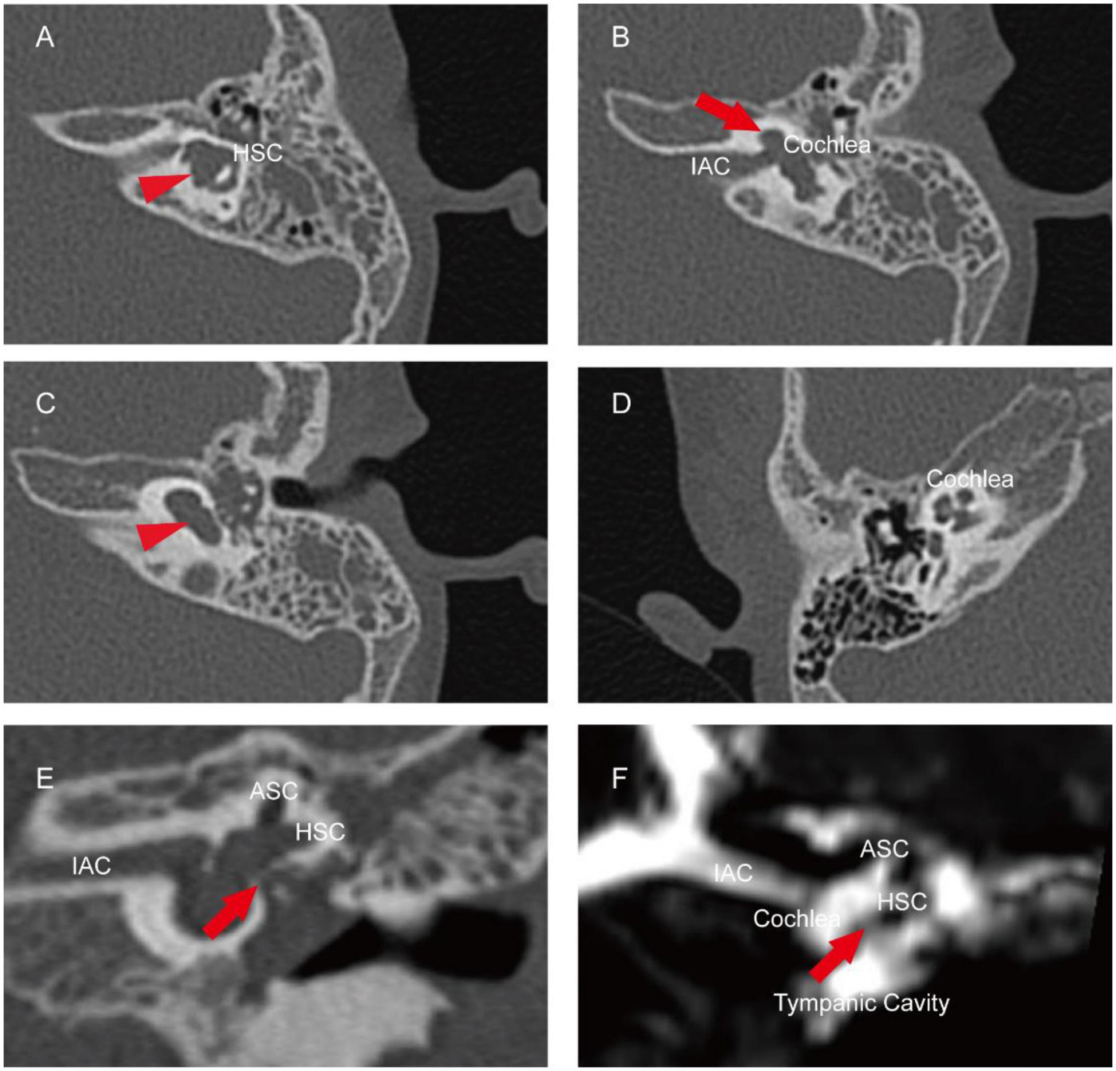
Preoperative imaging findings. (A) CT image at the level of the horizontal semicircular canal. The red arrowhead indicates an malformed HSC. (B) CT image at the level of the cochlea. The red arrow indicates the absence of the modiolus within the malformed cochlea. (C) The red arrowhead indicates the malformed and enlarged vestibule. (D) CT image of the normal right cochlea in the pediatric patient. (E) Coronal CT image of the tympanic cavity. The red arrow points to a bony discontinuity in the oval window region. Additionally, an isodense abnormal signal is present within the tympanic cavity. (F) Coronal T2-weighted MR image corresponding to panel E. The red arrow indicates an abnormal fluid communication between the cochlea and the tympanic cavity. The tympanic cavity is also filled with water signal. HSC, horizontal semicircular canal; ASC, anterior semicircular canal; IAC, internal auditory canal.
